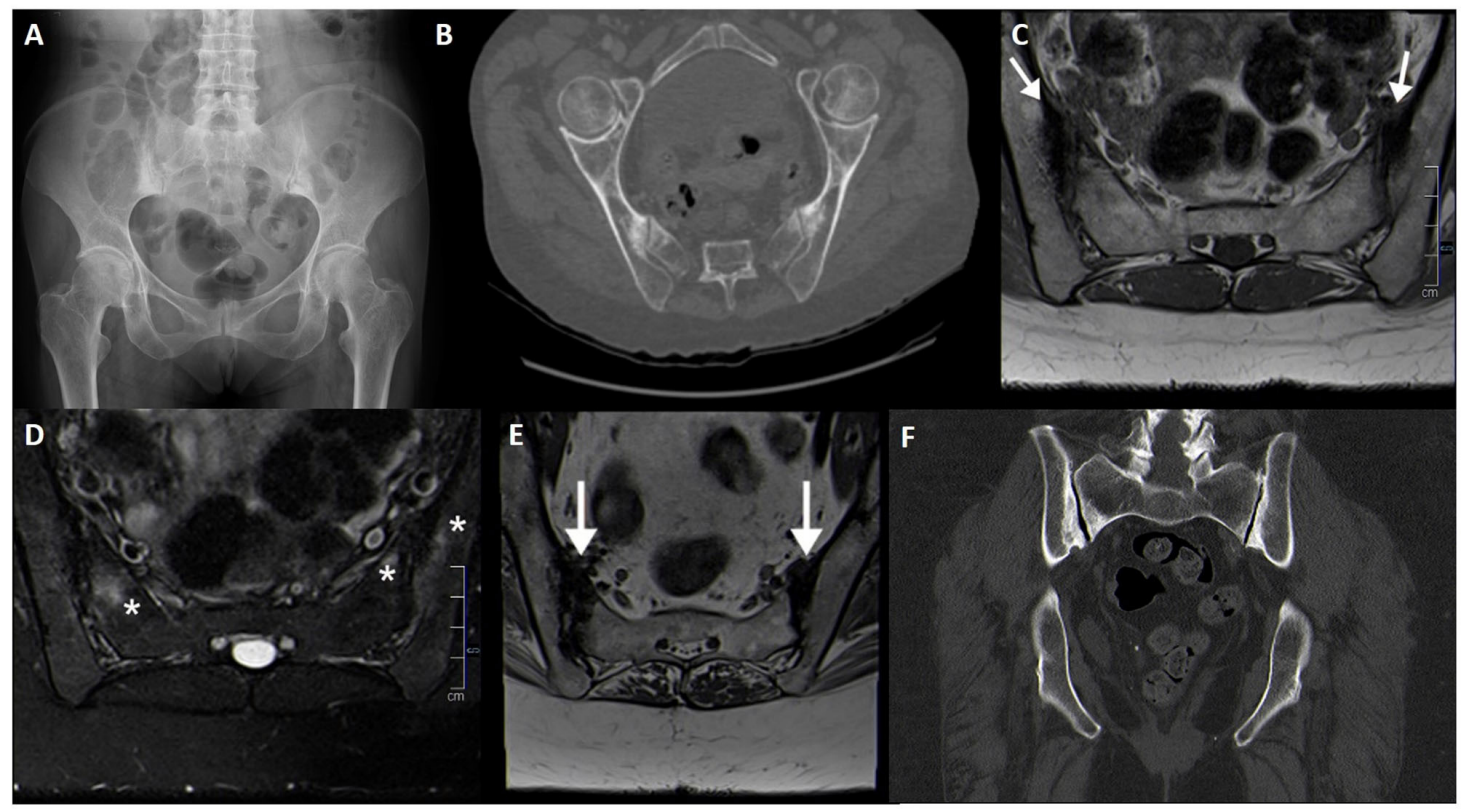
Fifty seven-year-old female patient with bilateral *osteitis condensans ilii* evident on pelvis radiography (A) and CT (B). Another patient, with post-partum bilateral bone marrow edema of the sacroiliac joint and sclerotic changes compatible with *osteitis condensans ilii* (arrows, asterisks), shown on MRI sequences (C–E) and CT (F).
